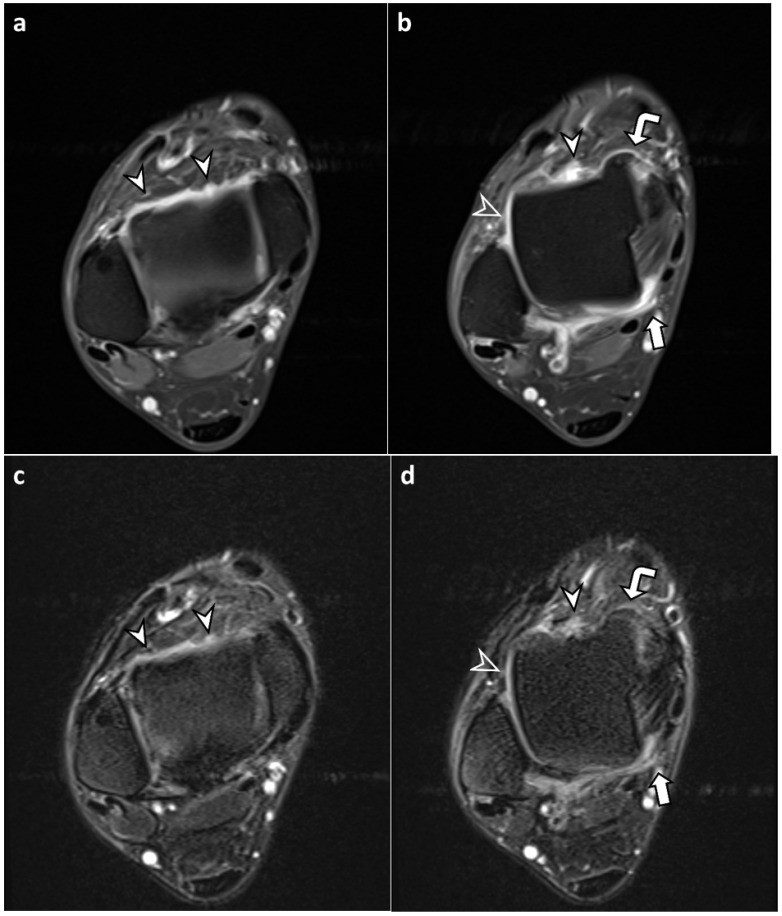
A 48-year-old male with chronic lateral ankle instability. (a,b) Axial contrast-enhanced, T1-weighted sequence (CE-T1) with fat suppression images reveal enhanced synovium in the anterior recess (arrowheads), anteromedial gutter (curved arrow), anterolateral gutter (open arrowhead), and posterior recess (arrow). Both readers assigned a synovial visibility grade of 4 (fully visible synovium with good tissue contrast) in all joint compartments. For synovial thickness score, reader 1 assigned 1 (maximum thickness 2–4 mm), 1, 0 (maximum thickness < 2 mm), and 2 (maximum thickness ≥4 mm) and reader 2 assigned 1, 2, 0, and 1 in the anterior recess, anteromedial gutter, anterolateral gutter, and posterior recess, respectively. (c,d) Corresponding axial fluid-attenuated inversion recovery sequence with fat suppression (FLAIR-FS) images in the same level reveal synovium showing hyperintense signal intensity, similar to CE-T1 images. Both readers assigned a synovial visibility grade of 4 in all joint compartments. For synovial thickness score, reader 1 assigned 1, 1, 0, and 2 and reader 2 assigned 1, 1, 0, and 1 in the anterior recess, anteromedial gutter, anterolateral gutter, and posterior recess, respectively.
